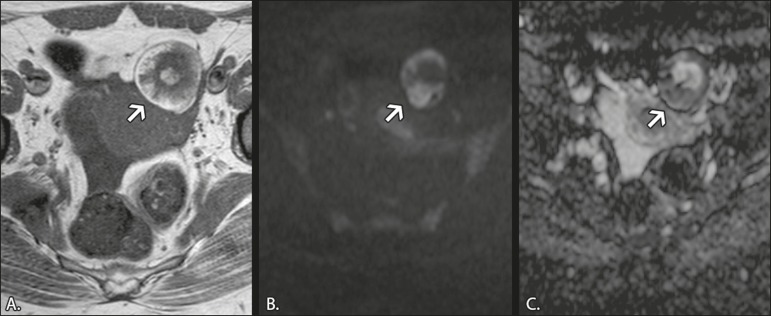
MRI of a 32-year-old female patient with a left mature cystic
